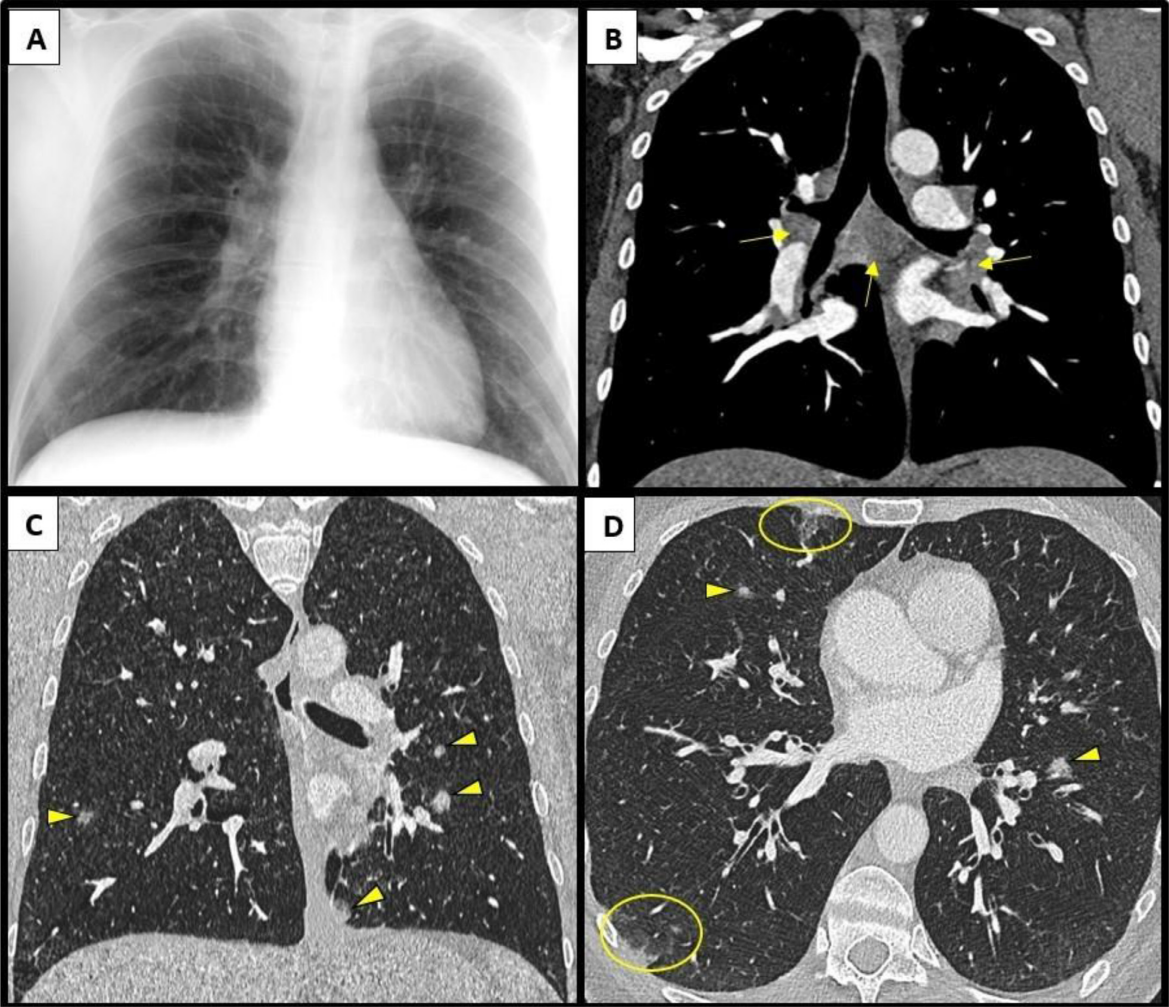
Chest radiograph and computed tomography images of the thorax. (A) Chest radiograph demonstrates faint multifocal nodular opacities within both lower zones and a bulky right hilum. (B) Coronal computed tomography image of the thorax in a soft tissue window confirms the suspected right hilar adenopathy and shows further adenopathy at the left hilum and within the mediastinum (arrows). (C) Coronal and (D) axial computed tomography images of the thorax in lung windows show multifocal nodular consolidation (arrow heads) and focal ground glass opacities (circled). Bibasal bronchial wall thickening is also seen in the axial images.
